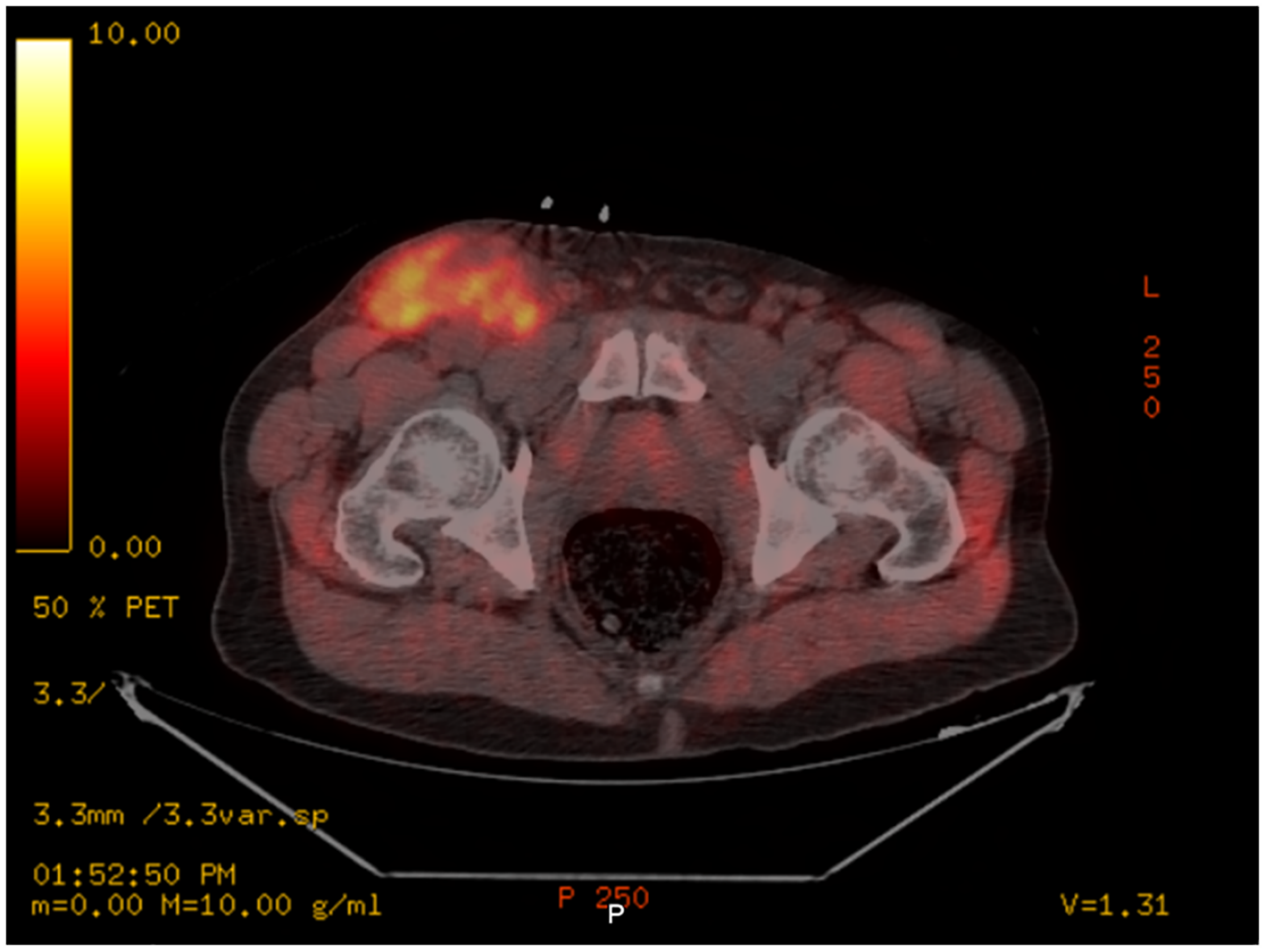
Axial PET/CT scan showing high fluorodeoxyglucose (FDG) uptake in the R inguinal node.
